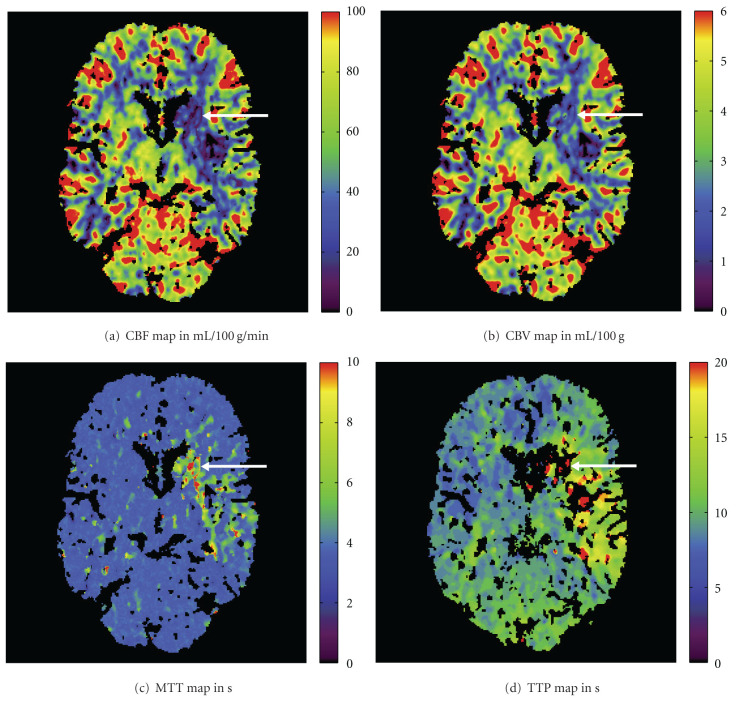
CT perfusion parameter maps of cerebral blood flow (CBF), cerebral blood volume (CBV), mean transit time (MTT), and time-to-peak (TTP). The ischemic stroke lesion is marked with arrows.
